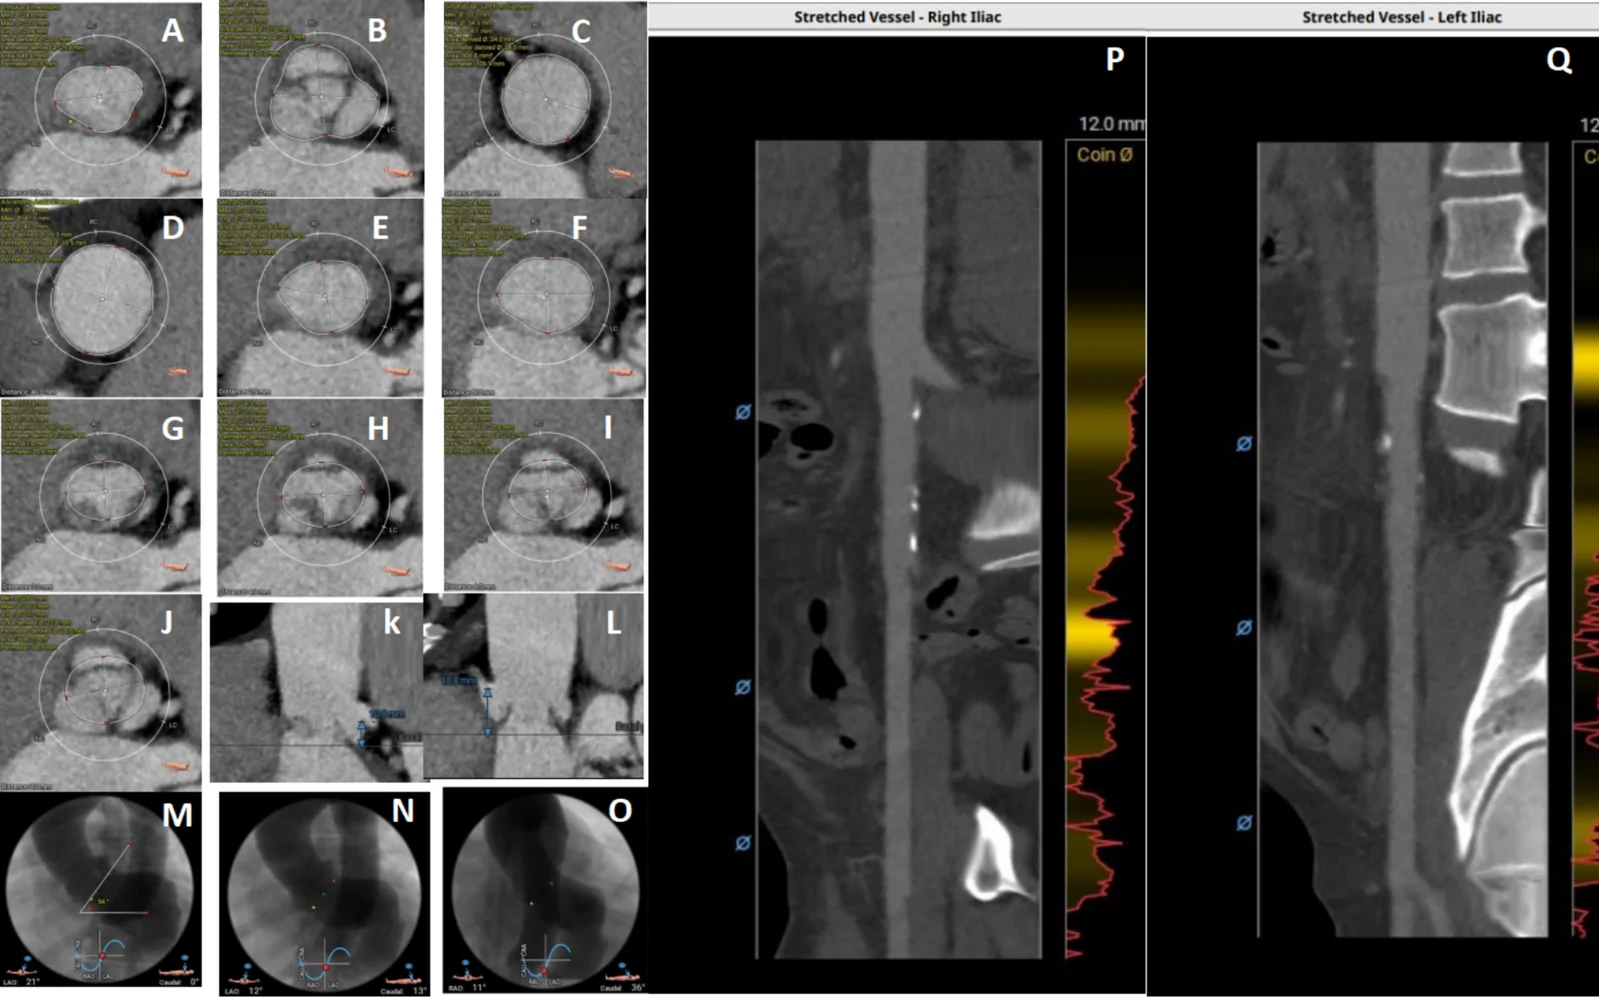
Preoperative 3-dimensional computed tomography (CT) evaluation of aortic root structure, virtual valve annulus angulation, cross-valve, valve release position, and transcatheter aortic valve replacement (TAVR) surgery path. The inner diameters of the aortic valve virtual annulus (A), sinus (B), sinus junction (C), and ascending aorta (D) were 29.8, 38.8, 34.0, and 39.9 mm, respectively. The inner diameters of the left ventricular outflow tract 2.5 and 5 mm below the plane of the virtual annulus (E and F) were 30.4 and 32.5 mm, respectively. The maximum inner diameters of supravalvular structures 2 (G), 4 (H), 6 (I), and 8 mm (J) above the plane of the virtual annulus were 27.2, 27.8, 27.2, and 28.0 mm, respectively. The height of the left coronary artery was 10.6 mm (K). The height of the right coronary artery was 18.8 mm (L). The results showed that the angle of the virtual valve ring was 54°, close to the standard of the transverse position (M). Middle position of the right coronary sinus (cross-valve position): left anterior oblique 12°, foot position 13° (N). Left and right coronary sinus overlapping position (valve release position): right anterior oblique 11°, foot position 36° (O). The right (P) and left (Q) femoral arteries had no obvious tortuosity or calcification, and the vessel diameter was >6.0 mm.
